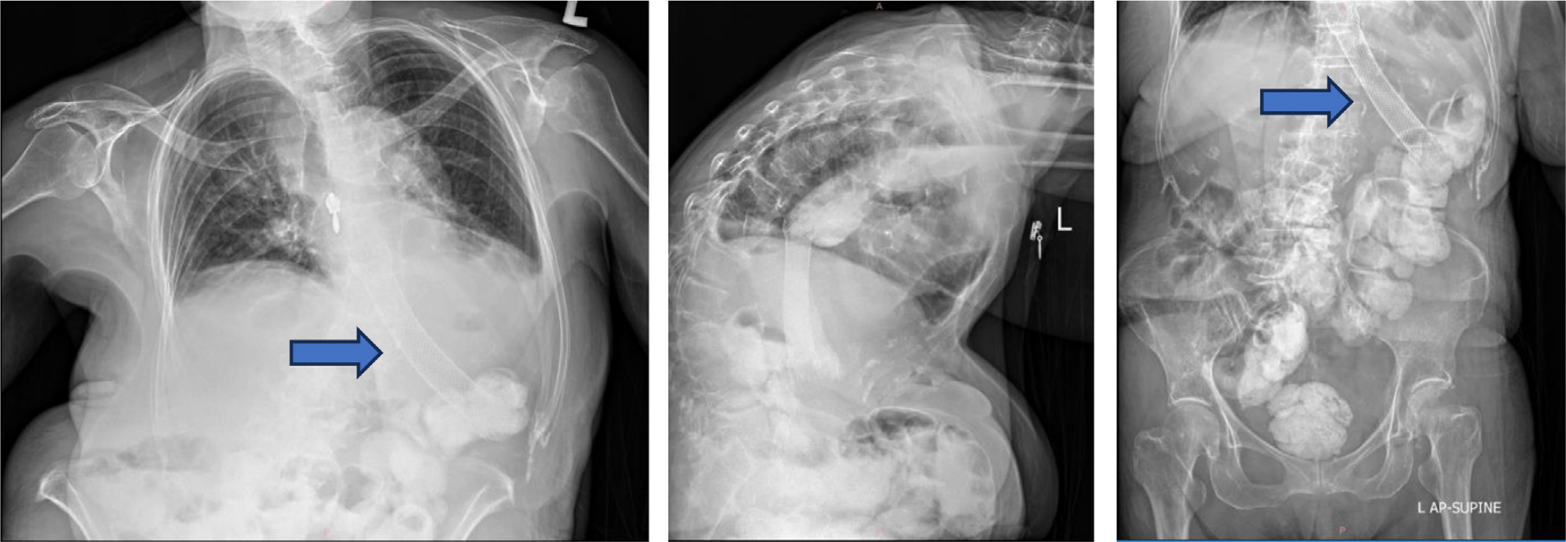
Esophageal stent shown on X-Ray imaging.
